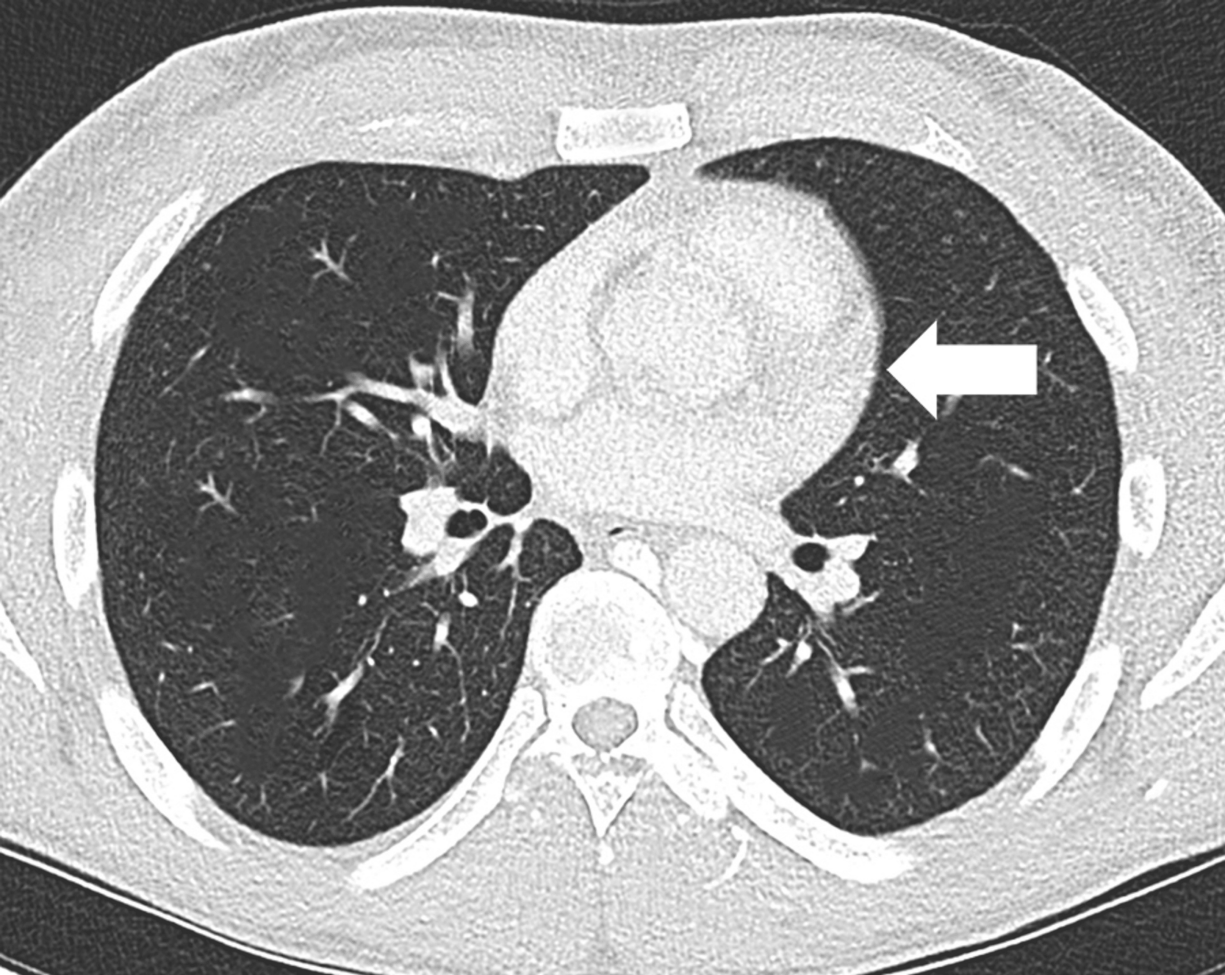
HRCT represents a large soft tissue mass in the anterior mediastinum, as pointed by the arrow. The mass appears to compress the superior vena cava. HRCT: high-resolution computed tomography
